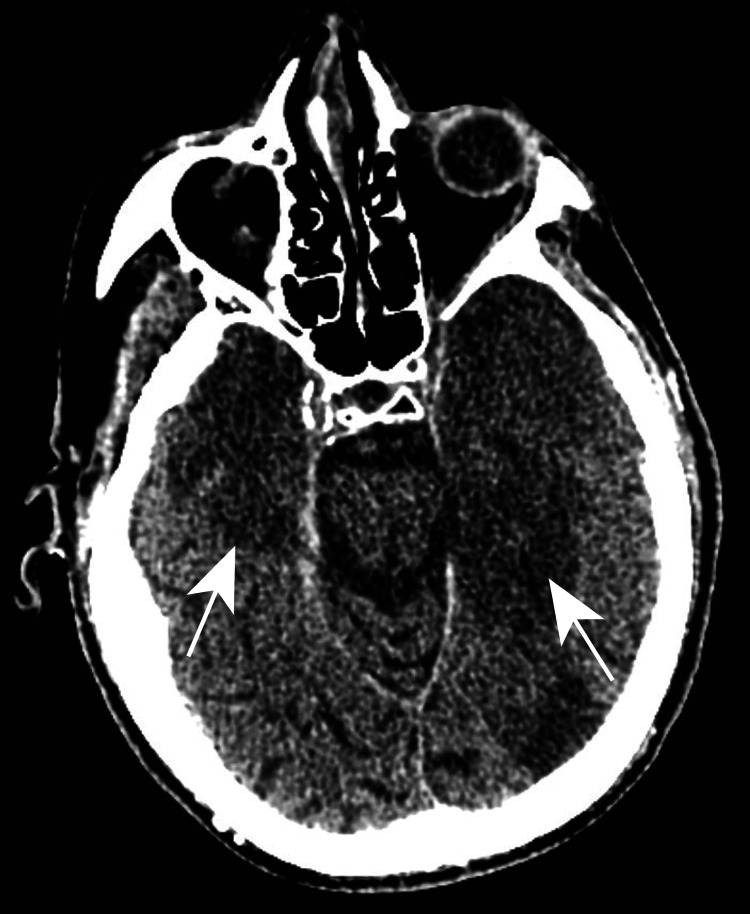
CT of the head with worsening hypoattenuation in the left temporal lobe and new right temporal lobe hypoattenuation
